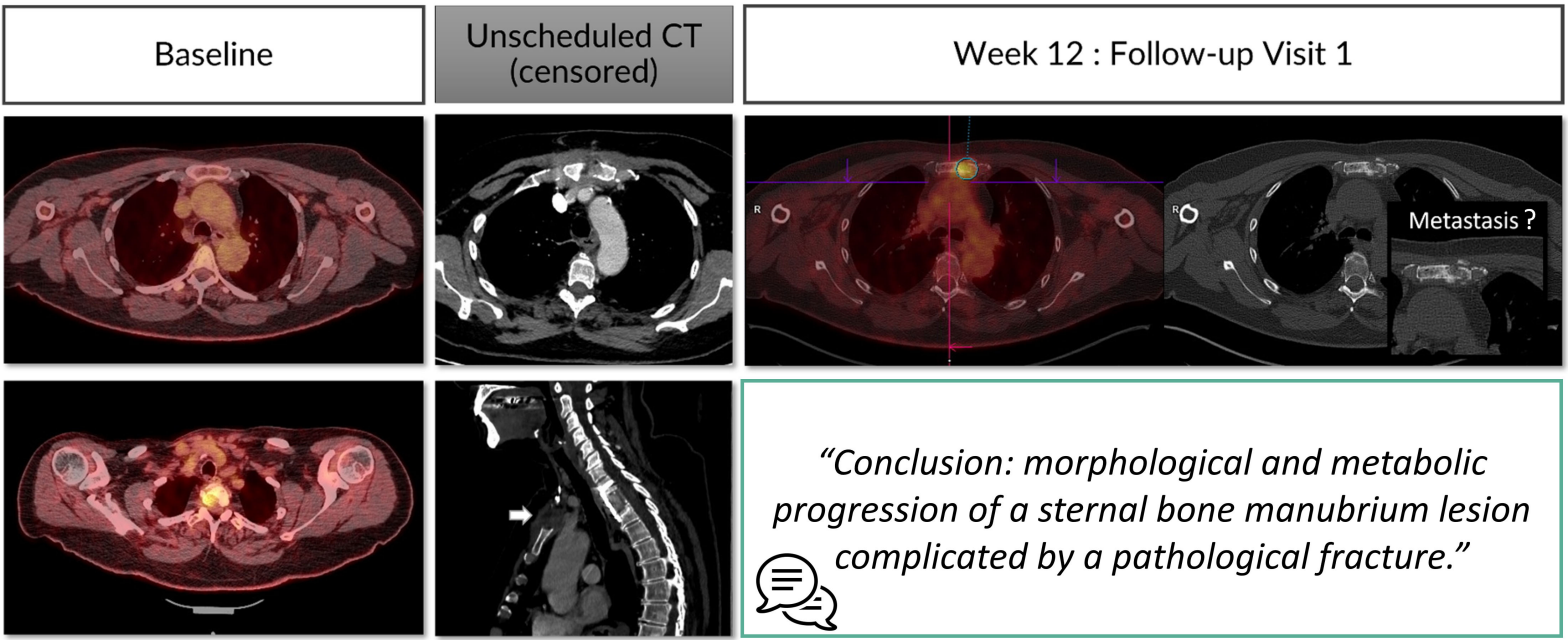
Type D error: misinterpretation linked to lacking prior information. A 53 year’s old patient is referred for a melanoma cancer follow-up with PET-CT. At the first follow-up visit, the radiologist was not aware of the metastatic vertebral stabilization with anterior sternotomy. This censored information misled the radiologist into finding a sternal lytic lesion that he thought was a metastasis.
